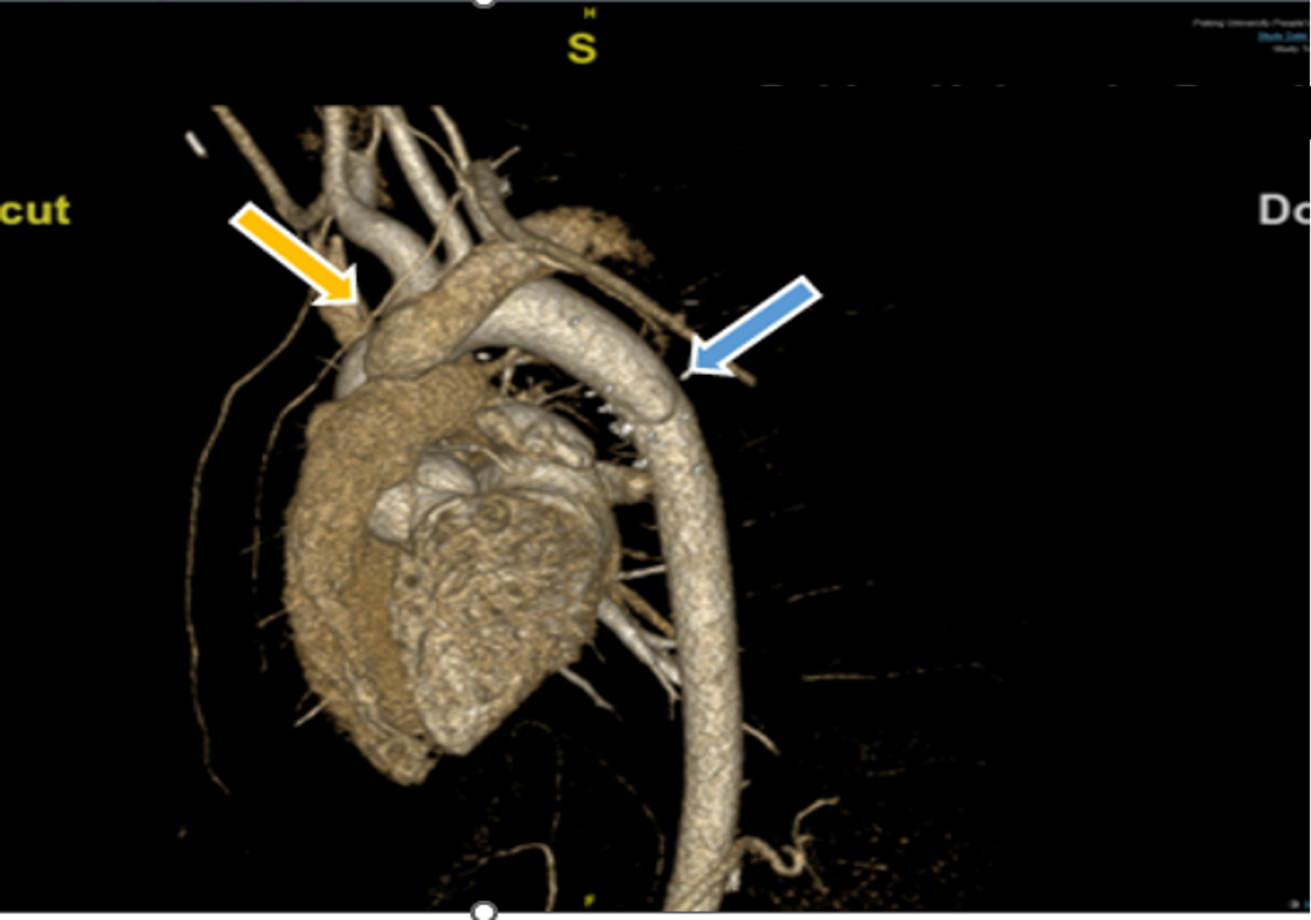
Postoperative volume-rendered cardiac CT angiography image. Repaired descending aorta with pericardium patch was showed as the blue arrow. AOLPA anastomosed to the main pulmonary artery *via* 18# synthetic vessel is indicated by the yellow arrow. AOLPA, anomalous origin of the left pulmonary artery; CT, computed tomography.
